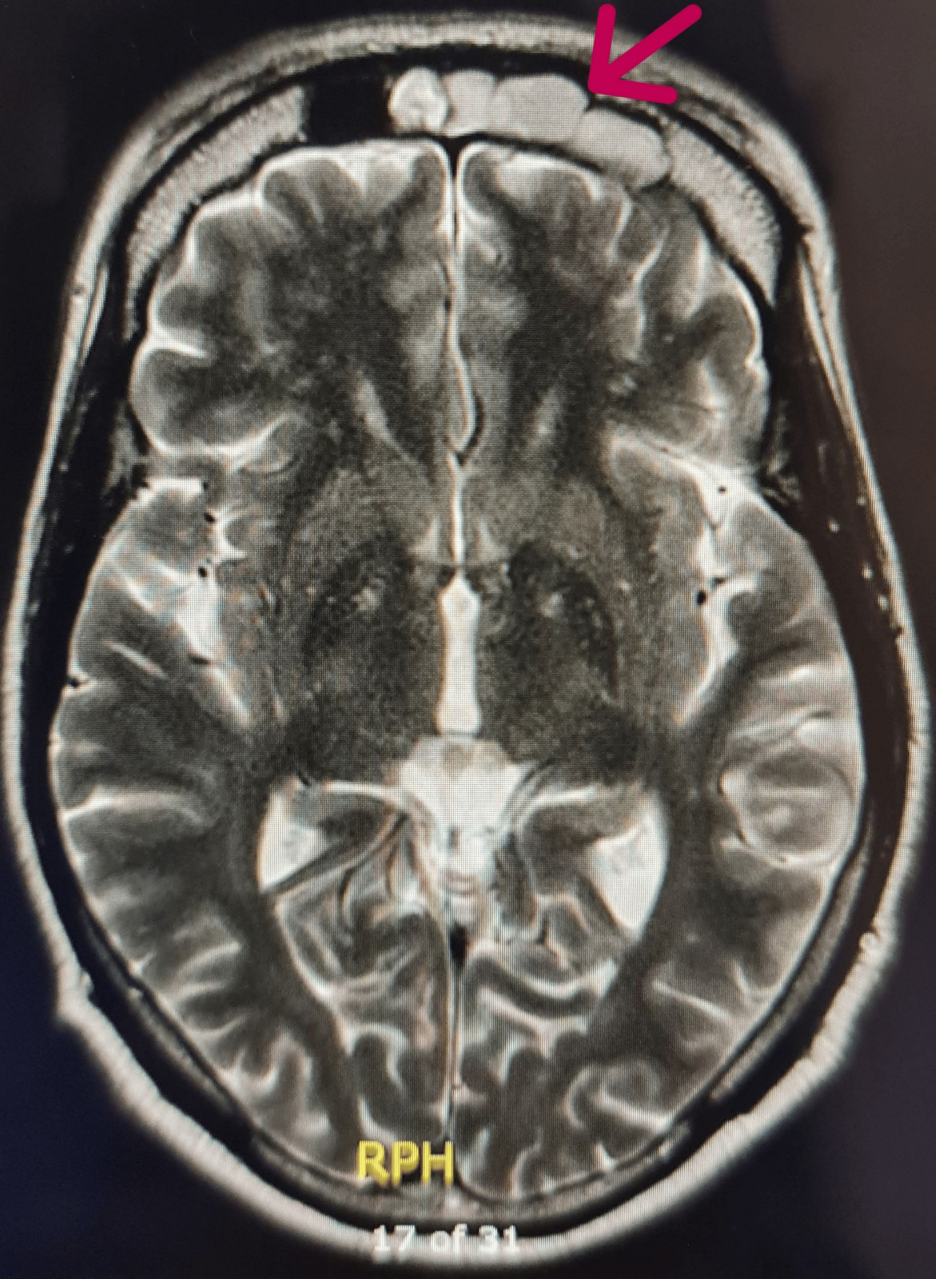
MRI brain (T2-weighted, axial view) showing intermediate signal intensity in the same left frontal bone lesion (arrow).
